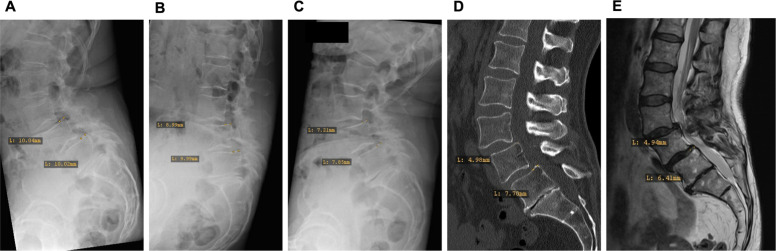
Anteroposterior vertebral body translation at L3/4 and L4/5 in (A) lateral standing neutral radiograph, (B) standing flexion radiograph, (C) standing extension radiograph, (D) horizontal computed tomography (CT), and (E) horizontal magnetic resonance imaging (MRI). There is no instability (translation ≥4 millimeters) in standing flexion/extension radiographs (B vs. C) or in standing neutral radiograph/horizontal CT (A vs. D) and MRI (A vs. E) at L4/5. However, instability can be seen in standing neutral radiograph/horizontal CT (A vs. D) and MRI (A vs. E) at L3/4.
